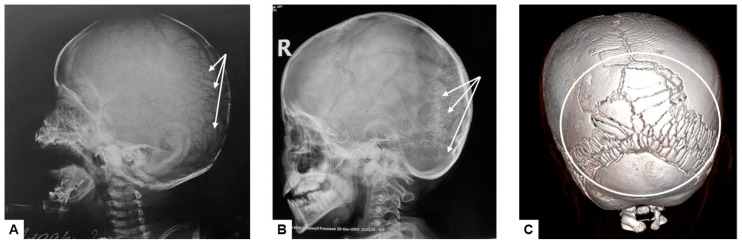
Abnormal ossification pattern of the skull vault: multiple wormian bones located in the region of lambdoid suture (white arrows on the lateral plane radiographs (A,B) and white circle on the reformed 3D CT image (C)).
